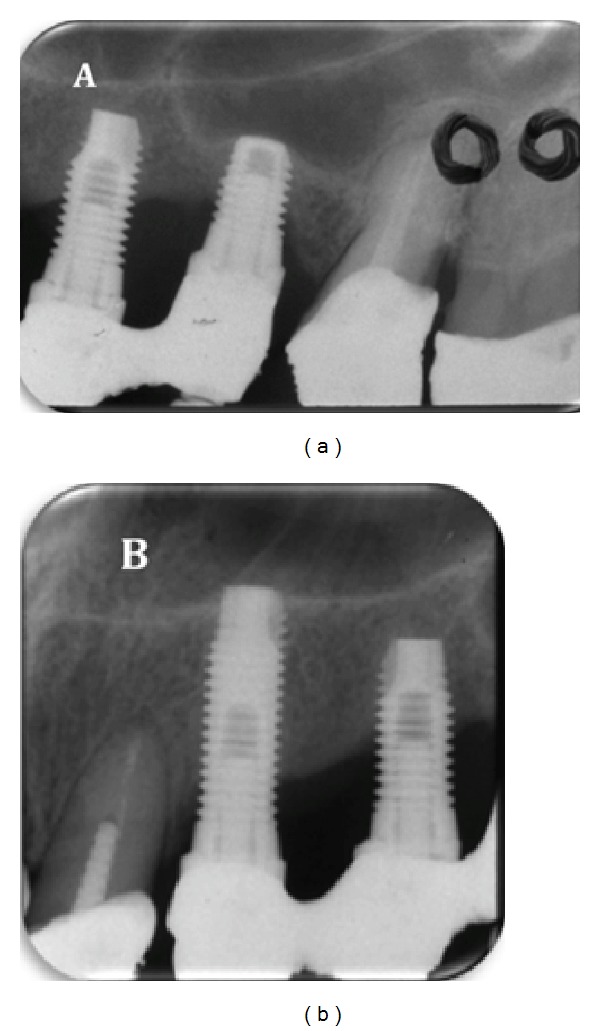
Radiographic initial appearance.
